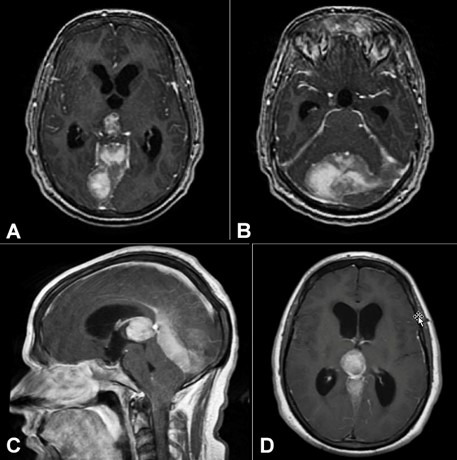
Brain magnetic resonance imaging (MRI). A – T1 axillary post-contrast images demonstrate a closely associated mass located parasagittal along the superior portion of the right tentorium and measuring 3.3 x 2.1 x 1.9 cm. Note the mass effect on the right occipital lobe; B – The T1 axial post-contrast shows that the enhancing lesion spans the midline within the posterior fossa and appears based within the tentorium and measures 4.5 x 6.1 x 1.8 cm; C – The sagittal; and D – axial post-contrast T1 isointense shows a heterogeneously enhancing mass of the tectum/pineal gland region, which measures 2.3 x 2.2 x 2.5 cm with pressure effect on the cerebellar hemispheres and tonsillar herniation of approximately 1.8 cm inferior to the cisterna magna.
